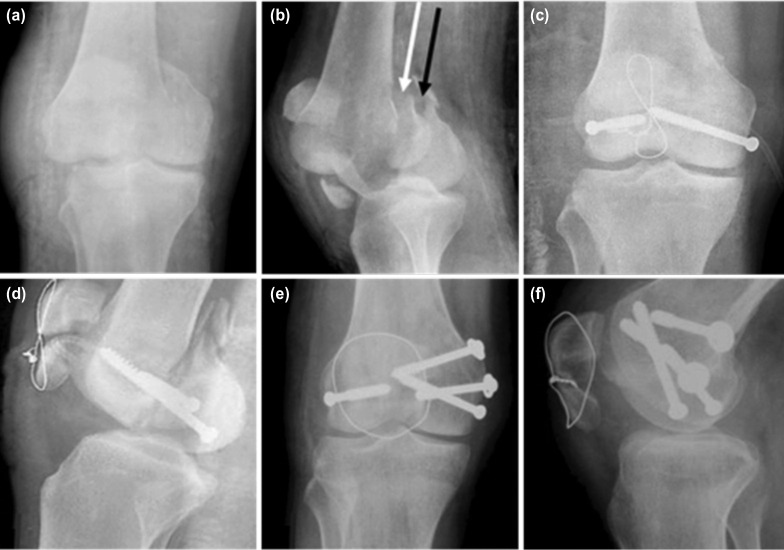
Radiographs at initial presentation, (a) Antero-posterior (AP) and (b) lateral view of the right knee showing the bicondylar Hoffa fracture (black and white arrow point to the larger medial condyle and small lateral condyle fragment respectively). Radiographs following anatomical reduction and stable internal fixation with 3 partially threaded 6.5mm cancellous lag screws with 2 washers for the medial condyle and 1 lag screw for the lateral condyle combined with a patella cerclage wire. Immediate postoperative (c) AP and (d) Lateral view of the right knee. (e) AP and (f) lateral view of the right knee at three months following surgery showing well united bicondylar Hoffa and patella fractures.
